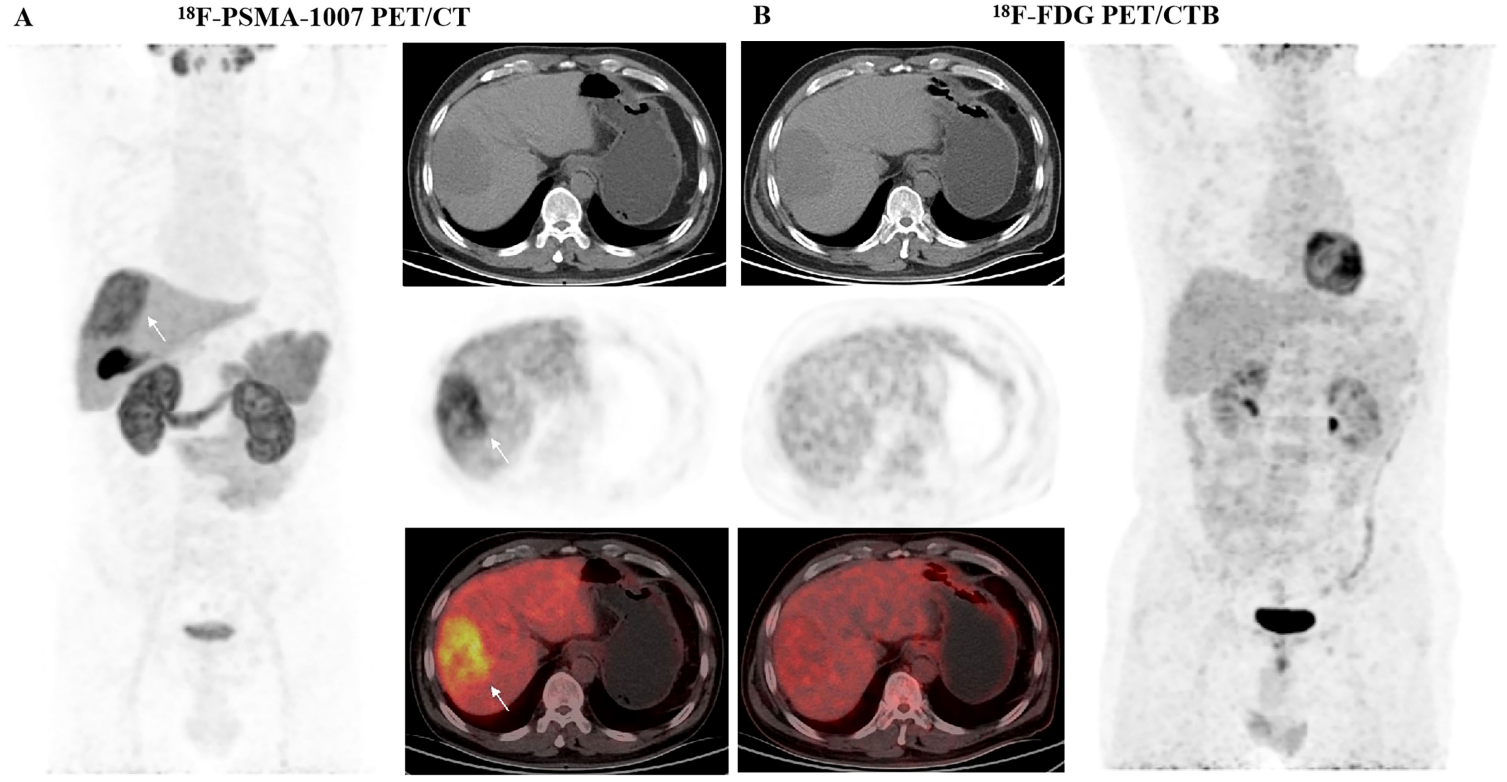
PET/CT images in a 54-year-old male patient with well-differentiated HCC. (A)^18^F-PSMA-1007 PET/CT revealed a strongly PSMA-avid lesion (white arrows, SUV_max_ = 20.3, T/L = 2.1) that was pathologically confirmed as well-differentiated HCC. (B)^18^F-FDG PET/CT showed no elevated uptake in the low-density mass of the liver right lobe (SUV_max_ = 4.6; T/L = 1.2).
